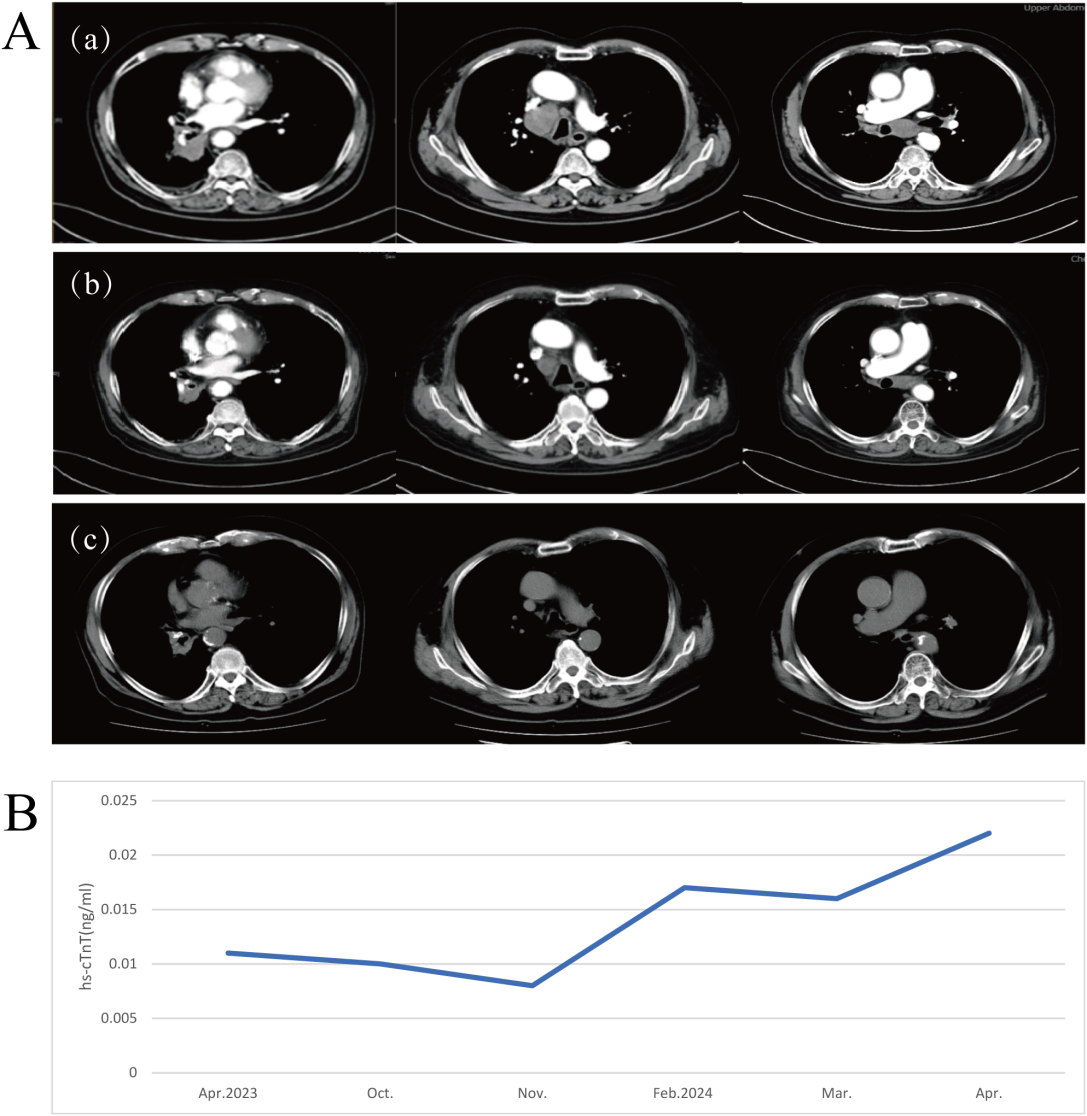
(A) Radiographic evaluation of cadonilimab as second-line therapy following disease progression on pembrolizumab. (a) Chest CT performed on October 16, 2023, prior to initiating cadonilimab. (b) Chest CT conducted on December 11, 2023, after three cycles of cadonilimab. (c) Chest CT obtained on March 6, 2025, following 22 cycles of cadonilimab therapy. (B) A transient and mild elevation in serum high-sensitivity cardiac troponin T (hs-cTnT) levels was observed during treatment(reference range: 0–0.014 μg/L).
